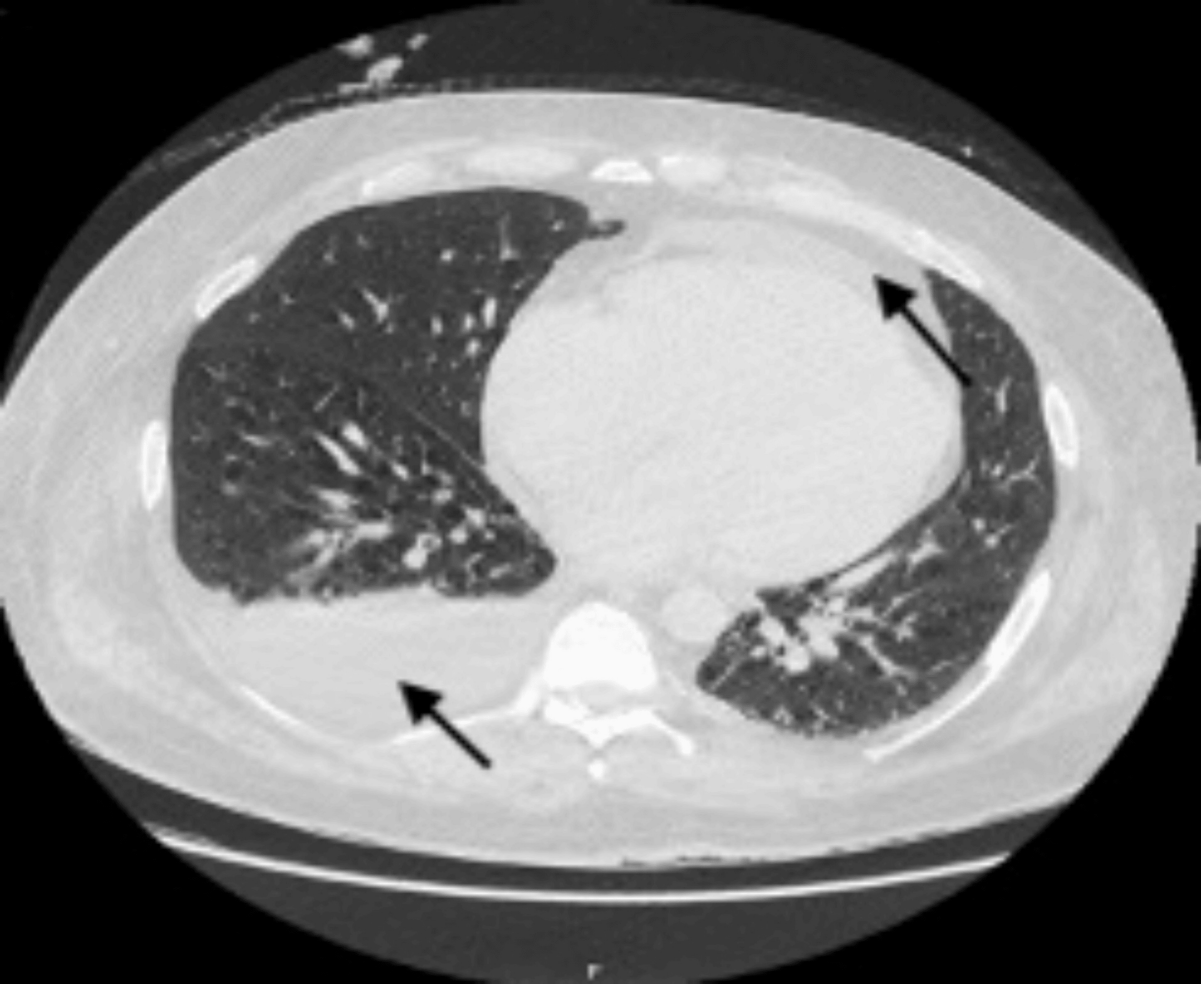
CT scan demonstrating right-sided pleural effusion and moderate pericardial effusion (indicated by arrows)
